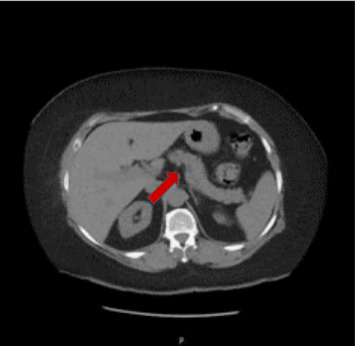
CT scan showing pancreatic edema and peripancreatic fat stranding.
